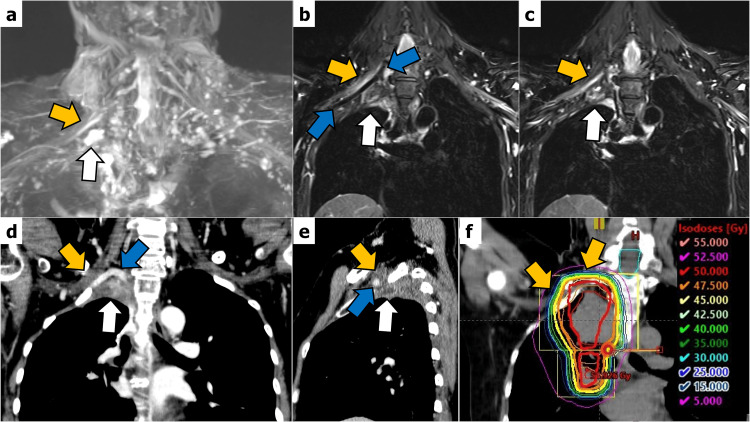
Comparison of postoperative brachial plexus evaluation and radiation planning CT images (a) In three-dimensional (3D) MR neurography, signal enhancement in the right brachial plexus (a, yellow arrow) and discontinuous fluid retention within postoperative scar tissue (a, white arrow) are depicted; (b, c) On the coronal 2D short tau inversion recovery (STIR) images, high signal intensity consistent with the right brachial plexus (yellow arrows) and postoperative scar tissue (white arrows) is observed, with the subclavian artery (blue arrows) intervening; (d, e) On contrast-enhanced CT, the lesion (yellow arrow) and postoperative scar tissue (white arrow) are separated by the right subclavian artery (blue arrows), confirming discontinuity; (f) Compared to the pre-treatment radiation planning CT, the abnormal signal in the brachial plexus corresponds to the area receiving 95% of the prescribed dose of 50 Gy (yellow arrows).
